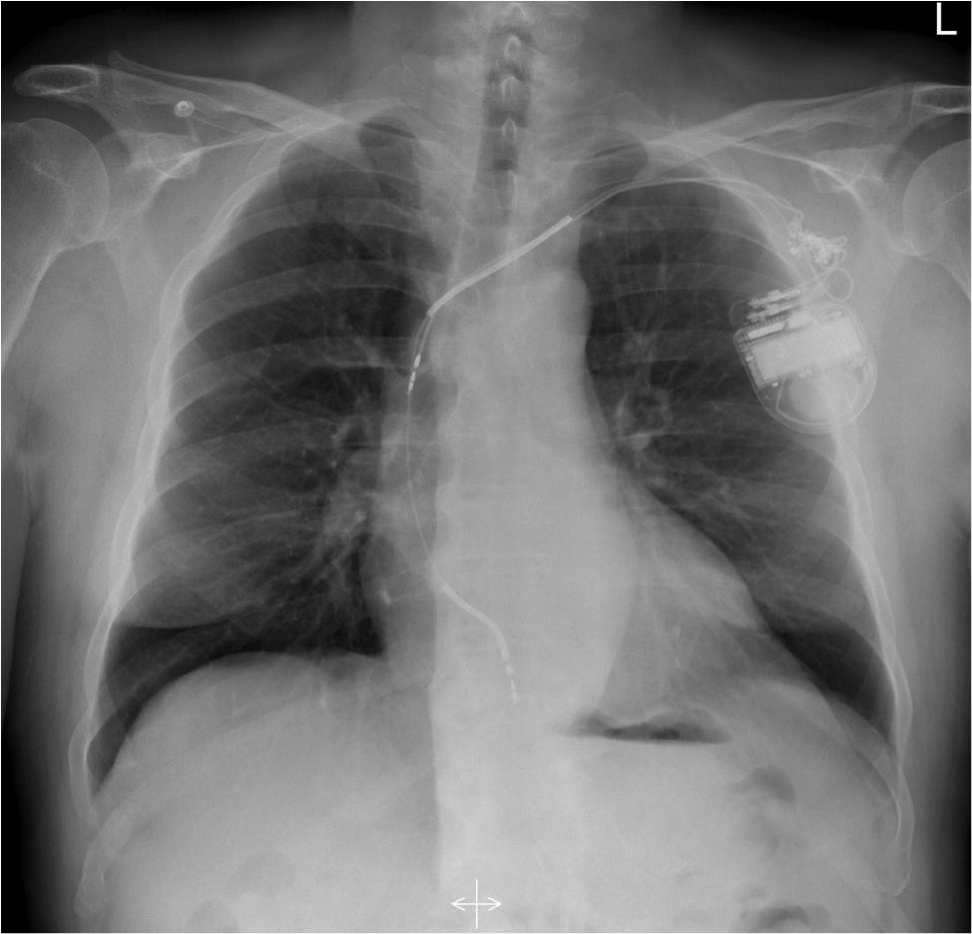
X-ray: dislodgement of the ventricular lead (projected to the area of the tricuspid annulus) and the atrial lead (tip projected to the superior vena cava)
